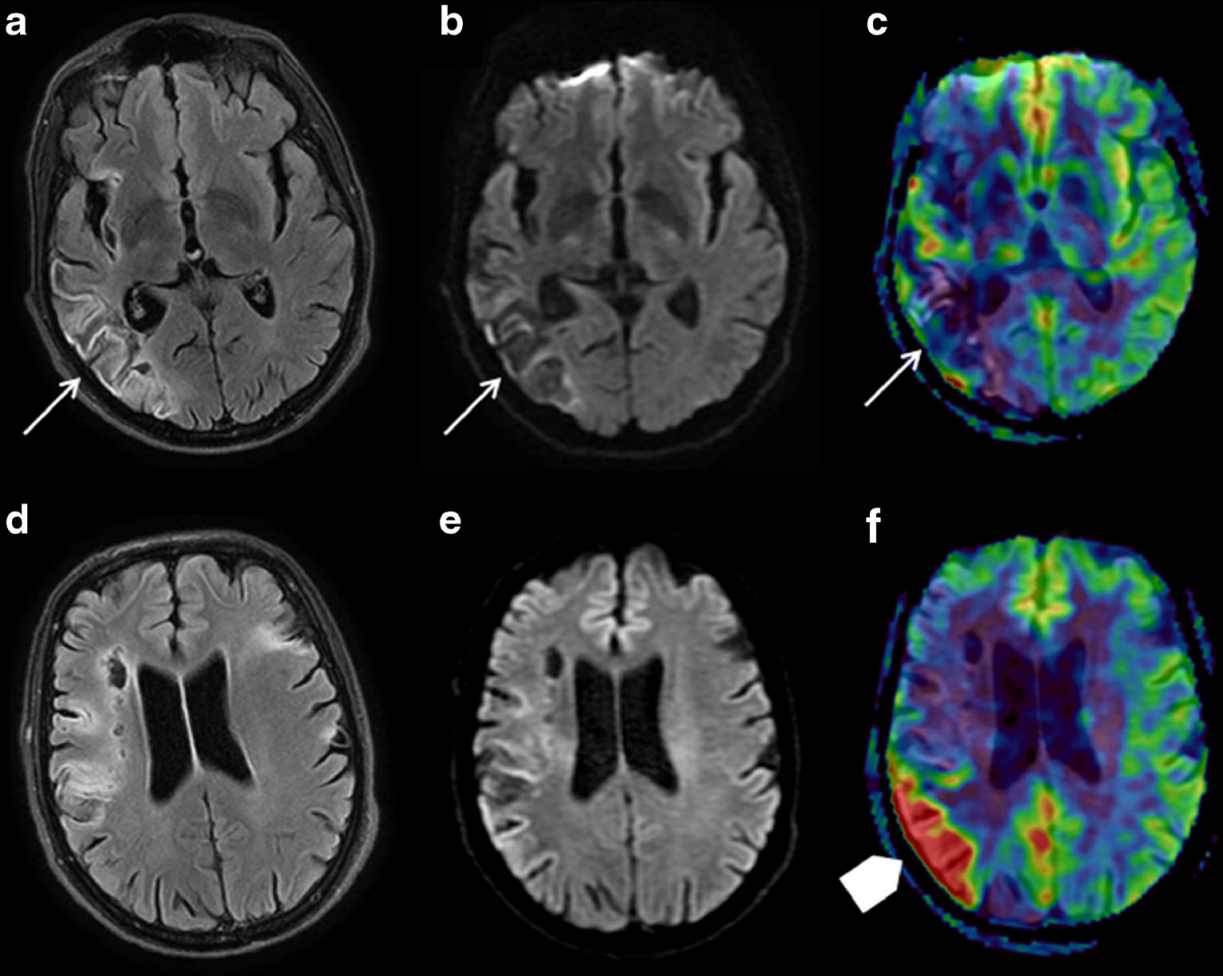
Brain MRI in an 85-year-old patient with a history of right sylvian ischaemic stroke presenting with recurrent left hemibody deficiency. FLAIR (a and d), DWI (b and e), and ASL/DWI fusion (c and f) sequences in the axial plane. a, b, c Sequelar right posterior sylvian zone with no sign of recent ischaemia (*white arrows*). d, e, f Hyperperfusion zone bordering the superior part of the cavity (*arrowhead*), without FLAIR or DWI anomaly, related to a partial seizure on ischaemic sequelae
